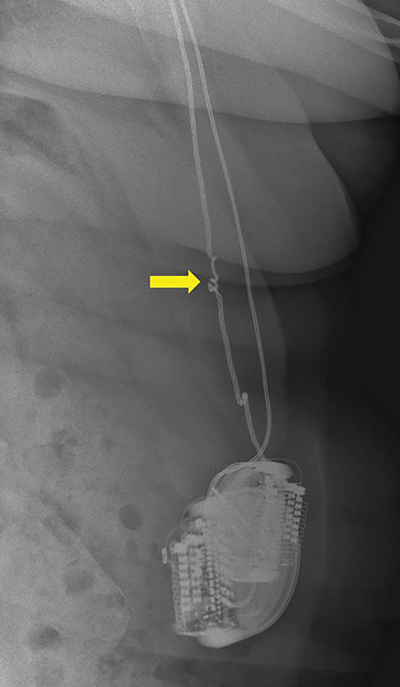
Abdominal x-rays show twisting of the neurostimulator and the electrodes around their axis, on the right side, with an interruption of the right electrode.
